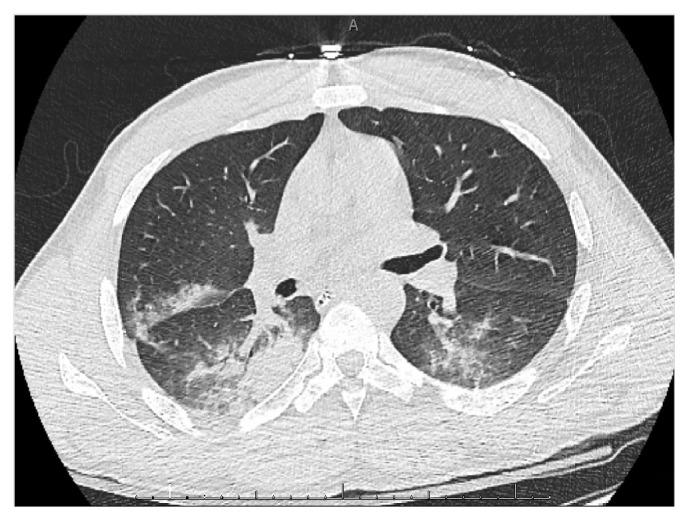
Computerized tomography (CT) of the chest 36 hours after admission, showing bilateral parenchymal opacities.
